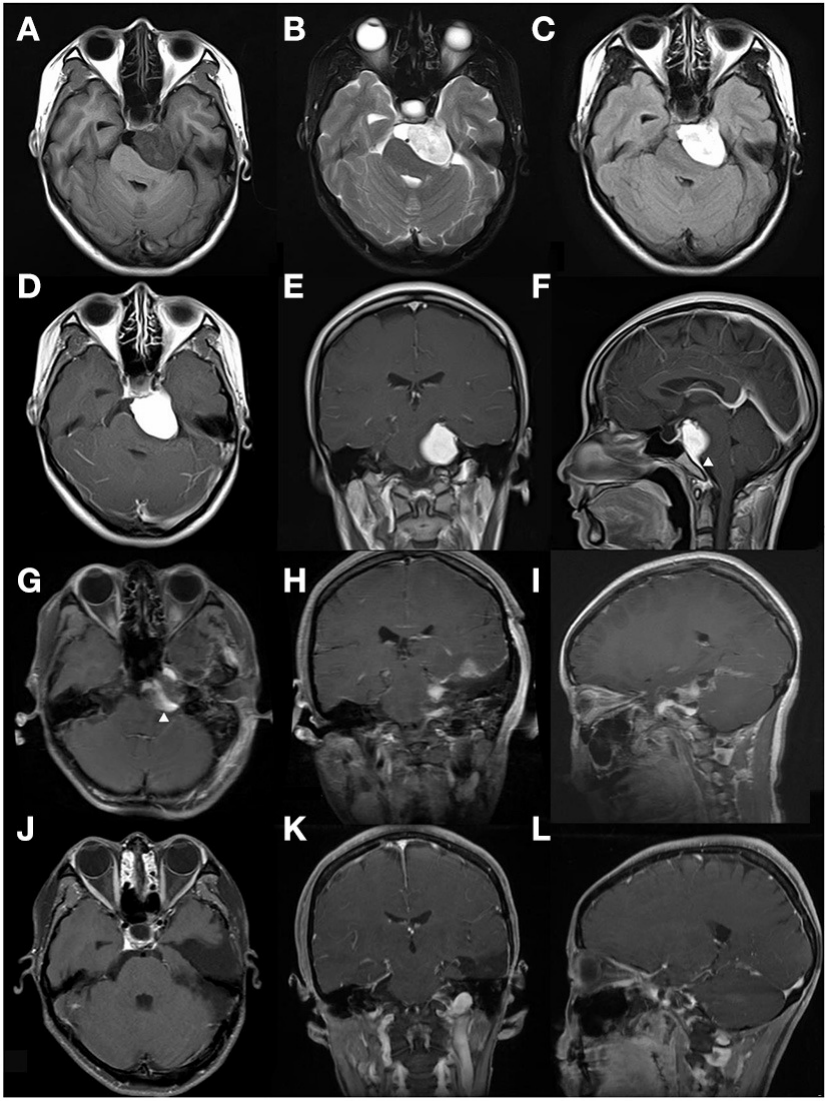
This case is a 26-year-old female patient (case 5 in Table 4). The tumor was found incidentally during a physical examination without any symptoms or signs. (A–C) Preoperative MRI scan showed a left CPA region tumor, defined as relatively heterogeneous signal intensity on T1, T2, and Flair-weighted MRI scan. The tumor shape was identified to be regular. (D–F) Enhanced MRI scan showed the lesion was a homogeneous enhancement. A dural tail sign was obviously evident arrowhead in (F). (G–I) On the 2nd day after the operation, an enhanced-MRI scan was performed and identified an incomplete resection arrowhead in (G). 10 days after surgery, this patient accepted radiotherapy (Gamma knife). (J–L) Enhanced MRI scan performed 8 years after surgery indicated that the residual tumor disappeared and no regression occurred.
